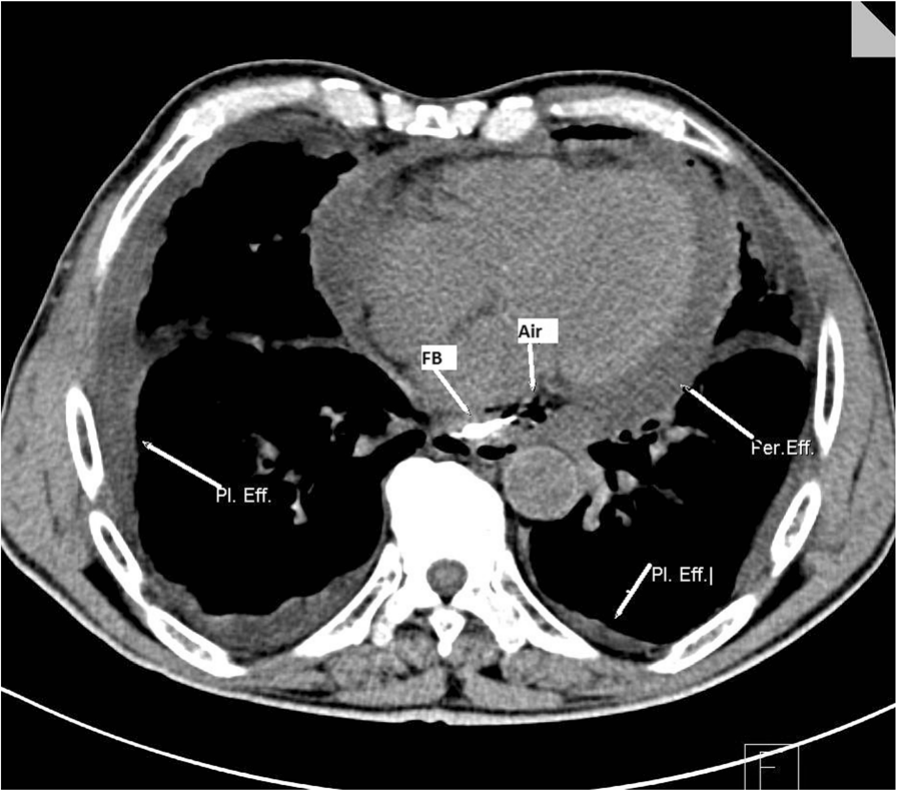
Findings of esophageal perforation by foreign body on computed tomography. Abbreviations: FB, Foreign body; Per. Eff., Pericardial effusion; Pl. Eff., Pleural effusion.
